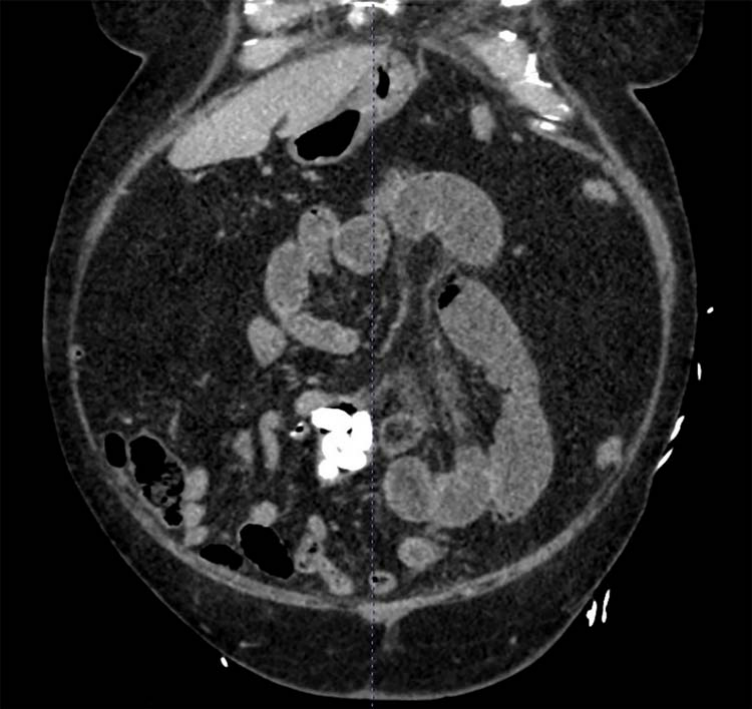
A CT scan of the abdomen and pelvis with contrast revealing a dilatation of the distal jejunum with air fluid levels and fecalization.
